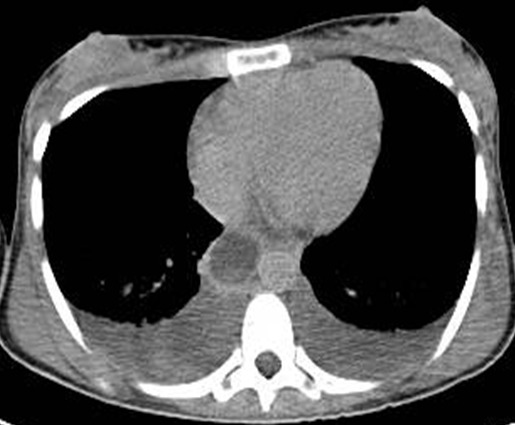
Axial CT scan showing hypo attenuated tumor thrombus in the inferior vena cava and bilateral pleural effusion
